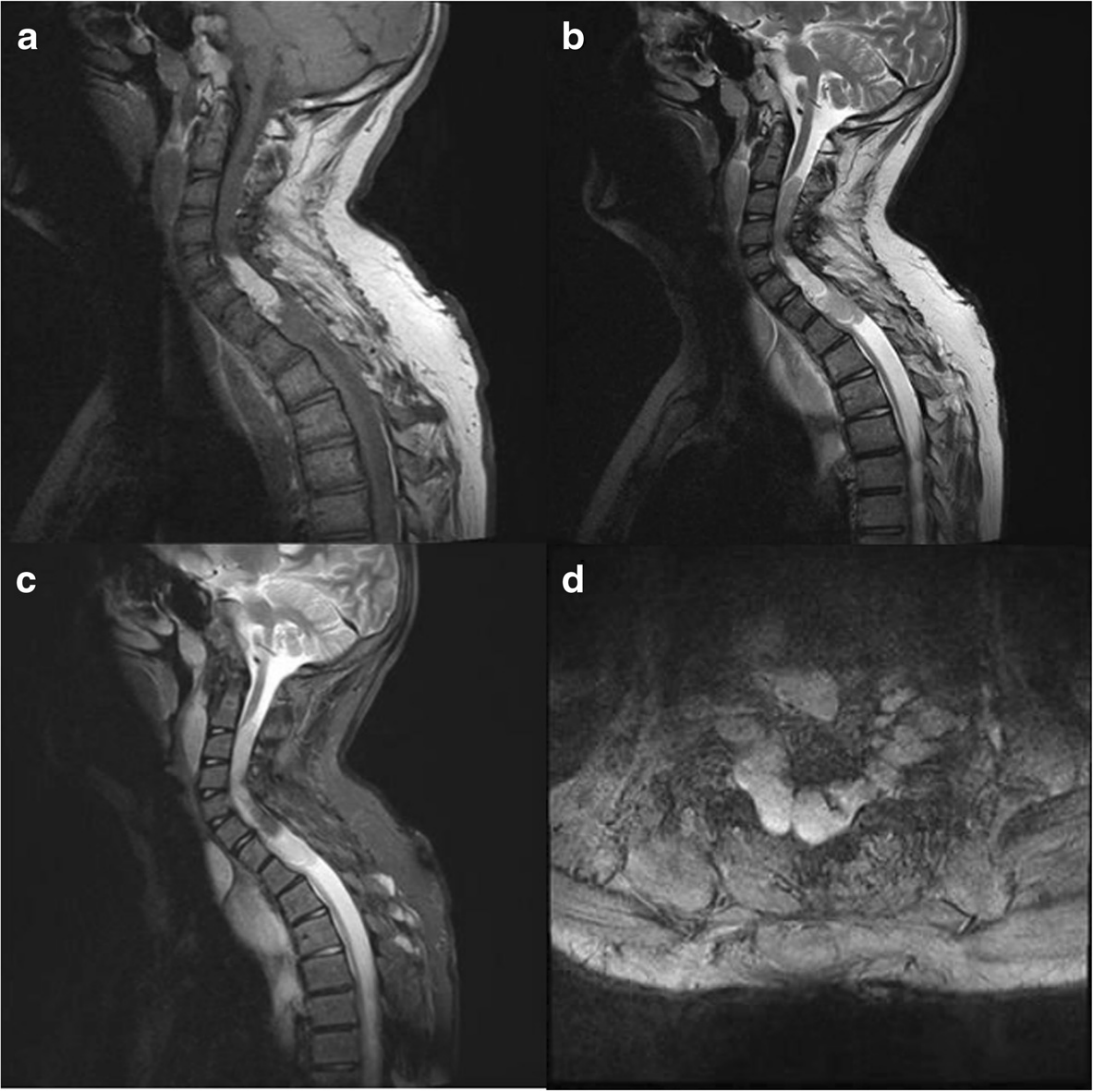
3T-MRI of the upper spine: T1 weighted sagittal image showing a large cervical and thoracic tumour mass (a). T2 weighted sequences (b) detect extensive intraspinal tumour formations, partially fat-isointense on fat-suppressed sequences (c). Transversal T2-images show the dimensions of the paravertebral mass (d).
